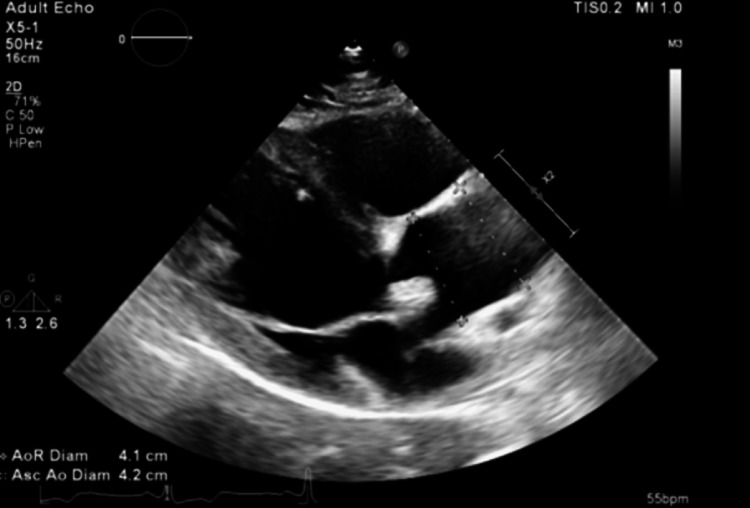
Dilation of the aortic root and proximal aorta depicted by the dotted lines X2 denotes a two-fold zoom applied by the echocardiography machine, indicating image magnification rather than an anatomical distance measurement.
